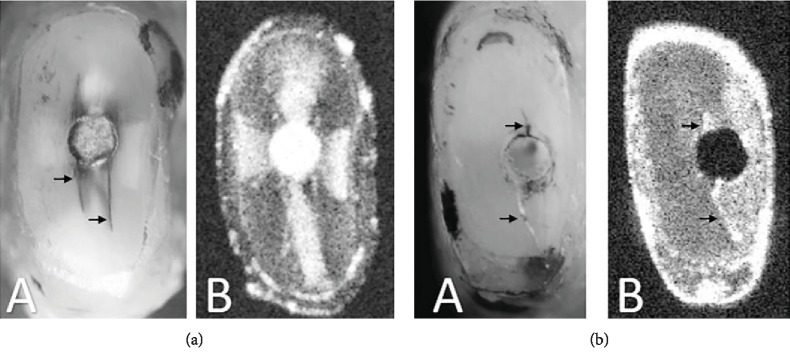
Representative images showing the same surface by DM (A) and OCT (B). (a) MTA group after two months of obturation. (b) Control group immediately after ultrasonic preparation. Arrows: crack lines.
